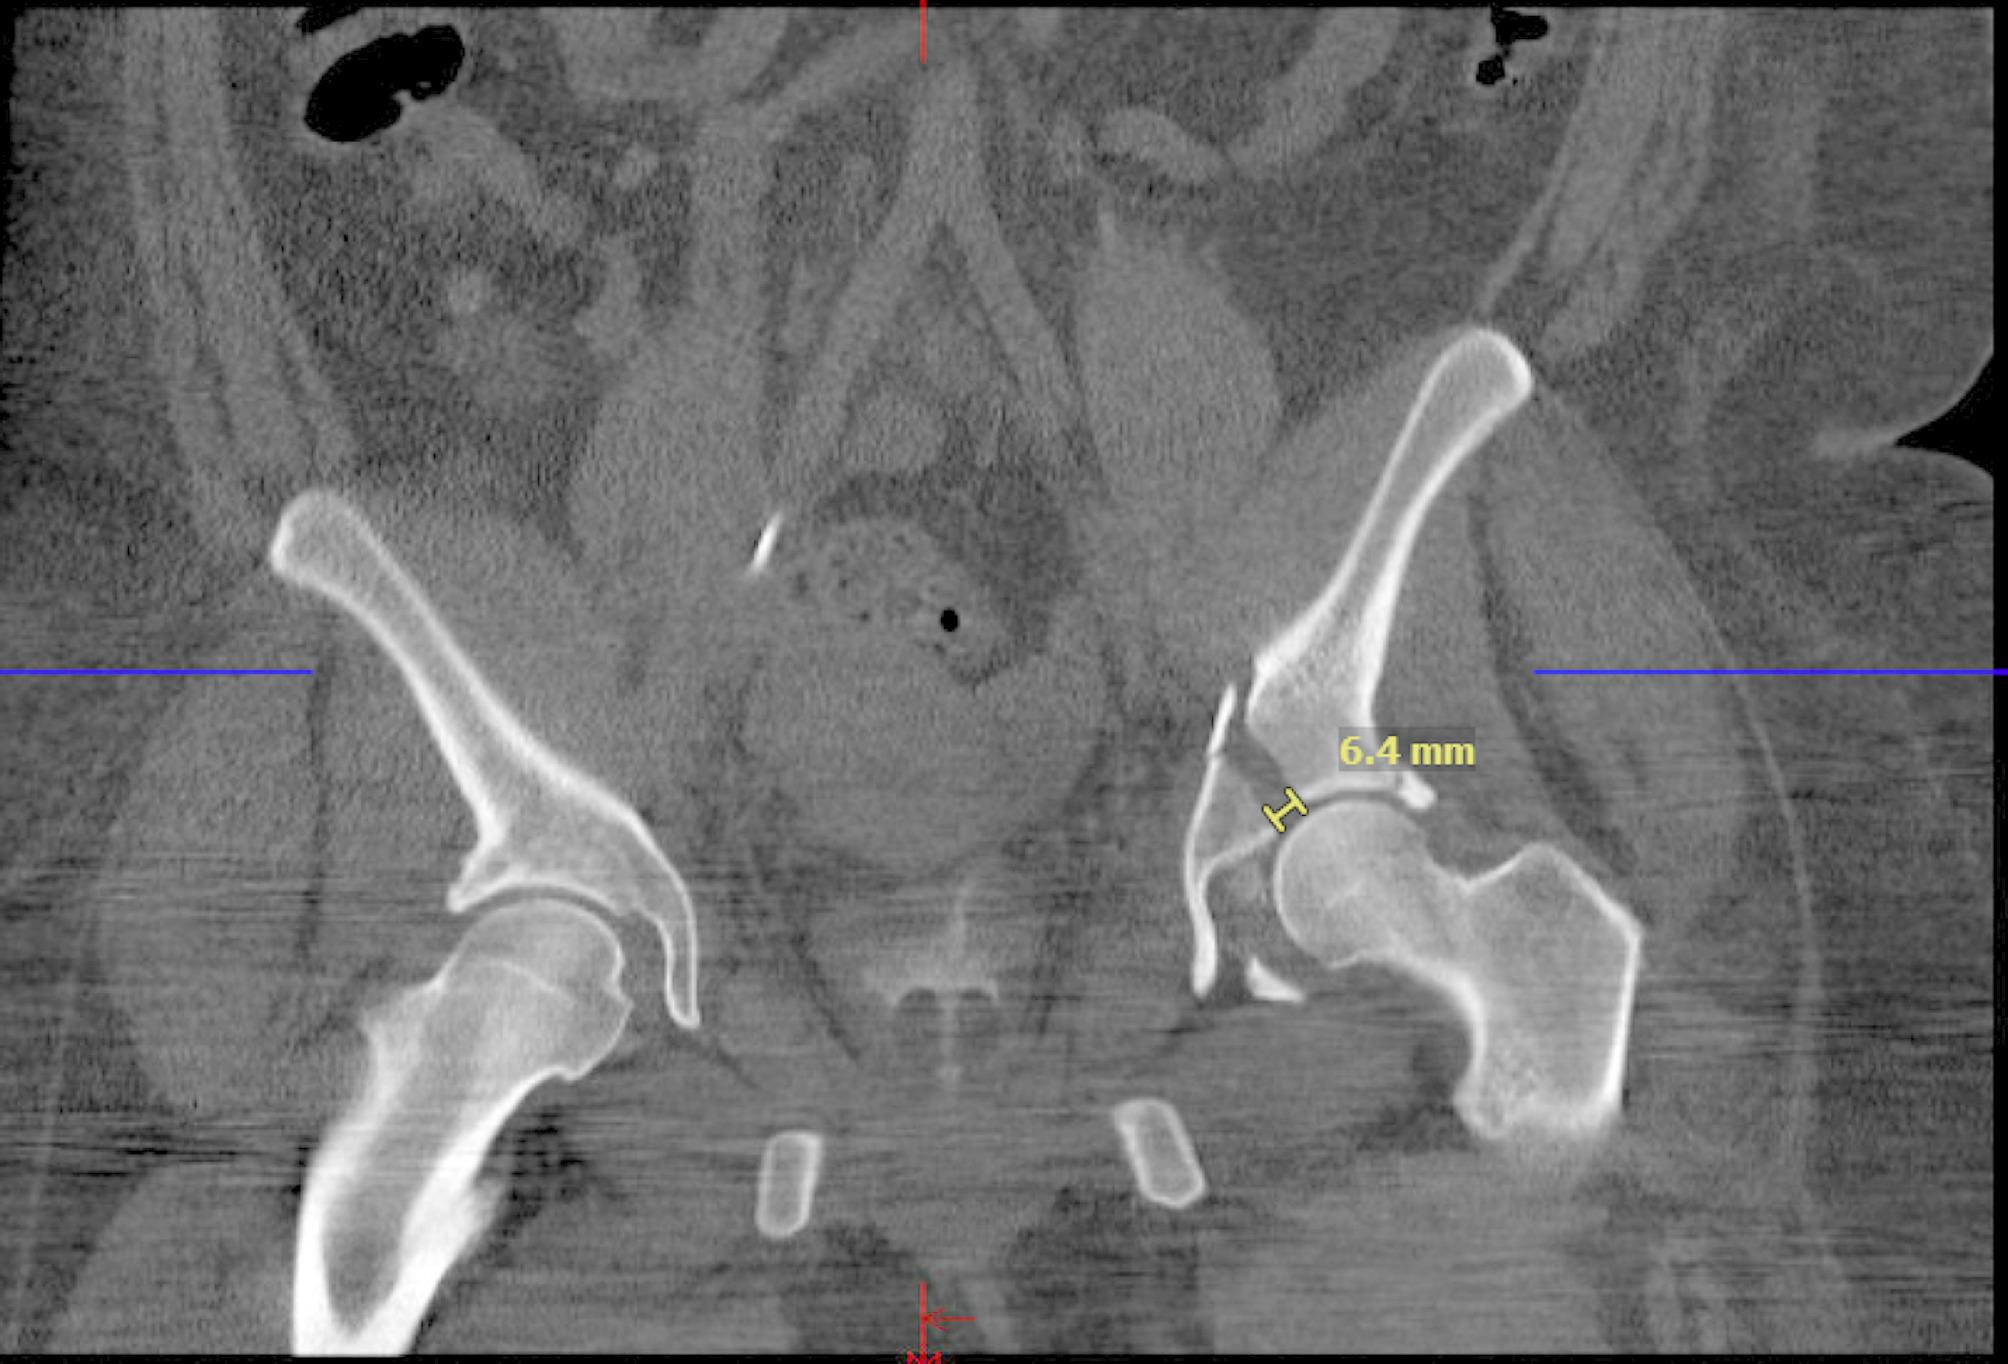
Max displacement of where the gap of the fracture is the largest in coronal computed tomography axis
